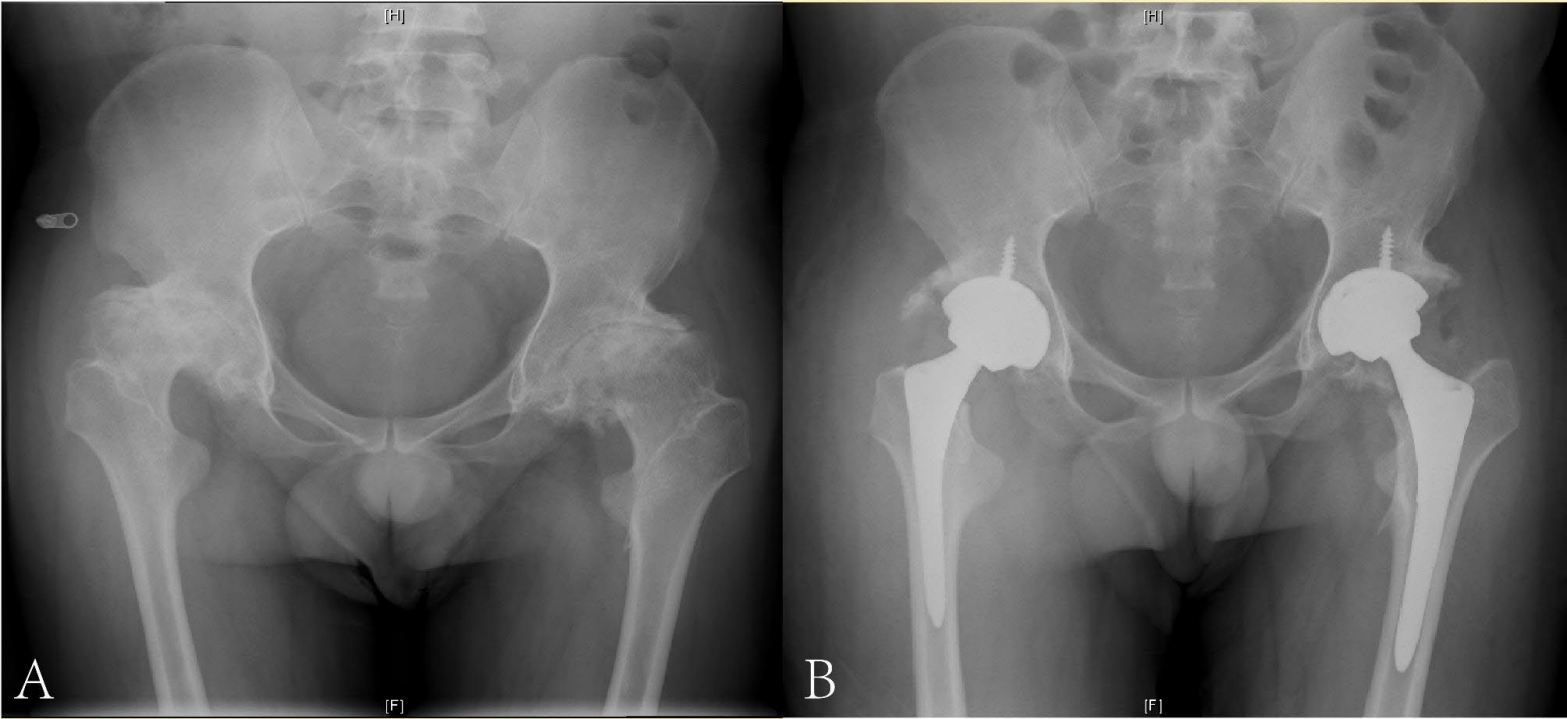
Radiographs of a male patient with advanced bilateral haemophilic hip arthropathy. (A) Preoperative anteroposterior view. (B) The latest follow up anteroposterior radiograph after total hip arthroplasty twice
